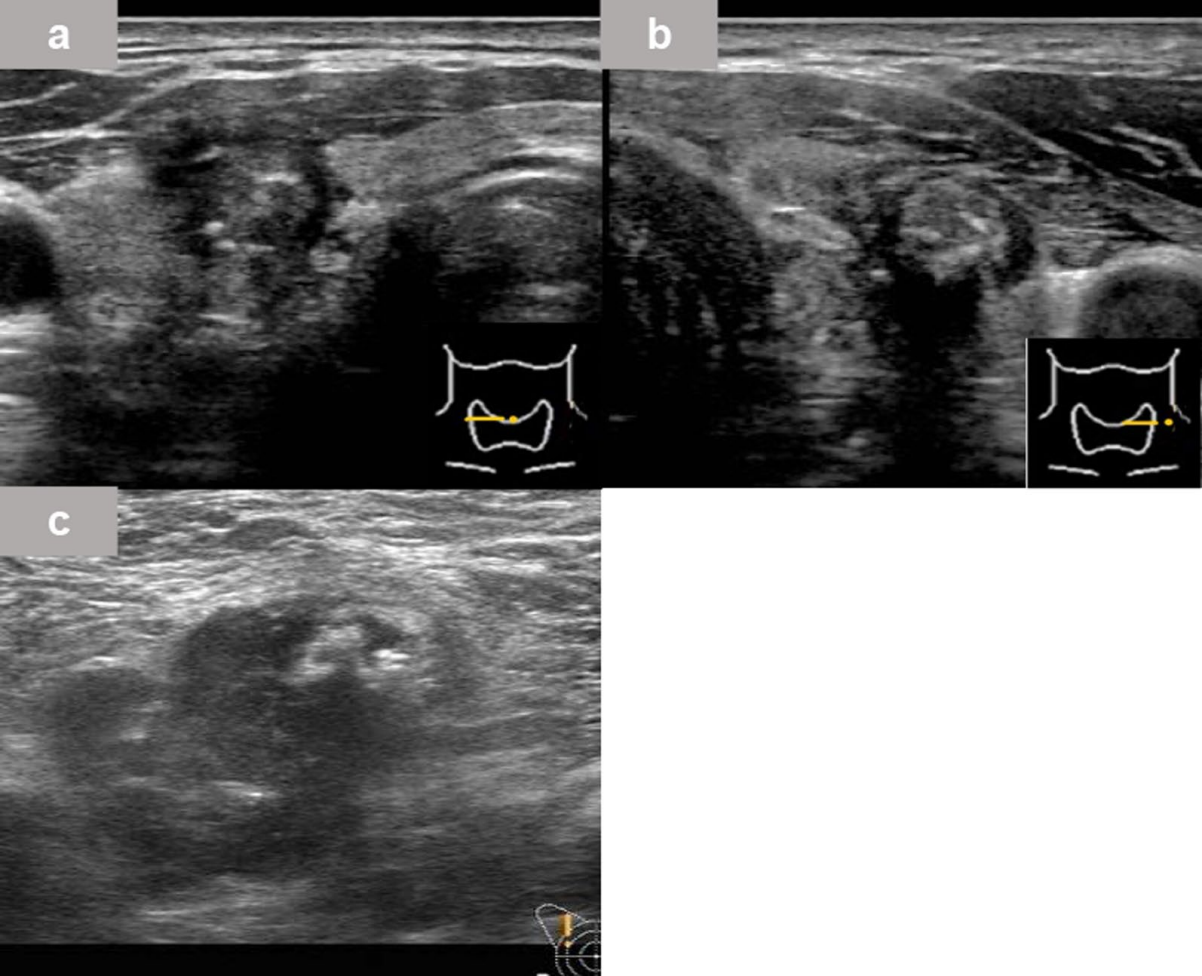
Ultrasonography findings. a, b Irregularly shaped, ill-defined, hypoechoic mass was seen in the right (a) and left (b) lobes of the thyroid gland. There were hyperechoic spots with an acoustic shadow. c Right axillary tumor was a hypoechoic tumor. It had a well-defined and rough border. It showed internal heterogeneity and its size was 30 mm. There was no obvious enlargement of surrounding lymph nodes
